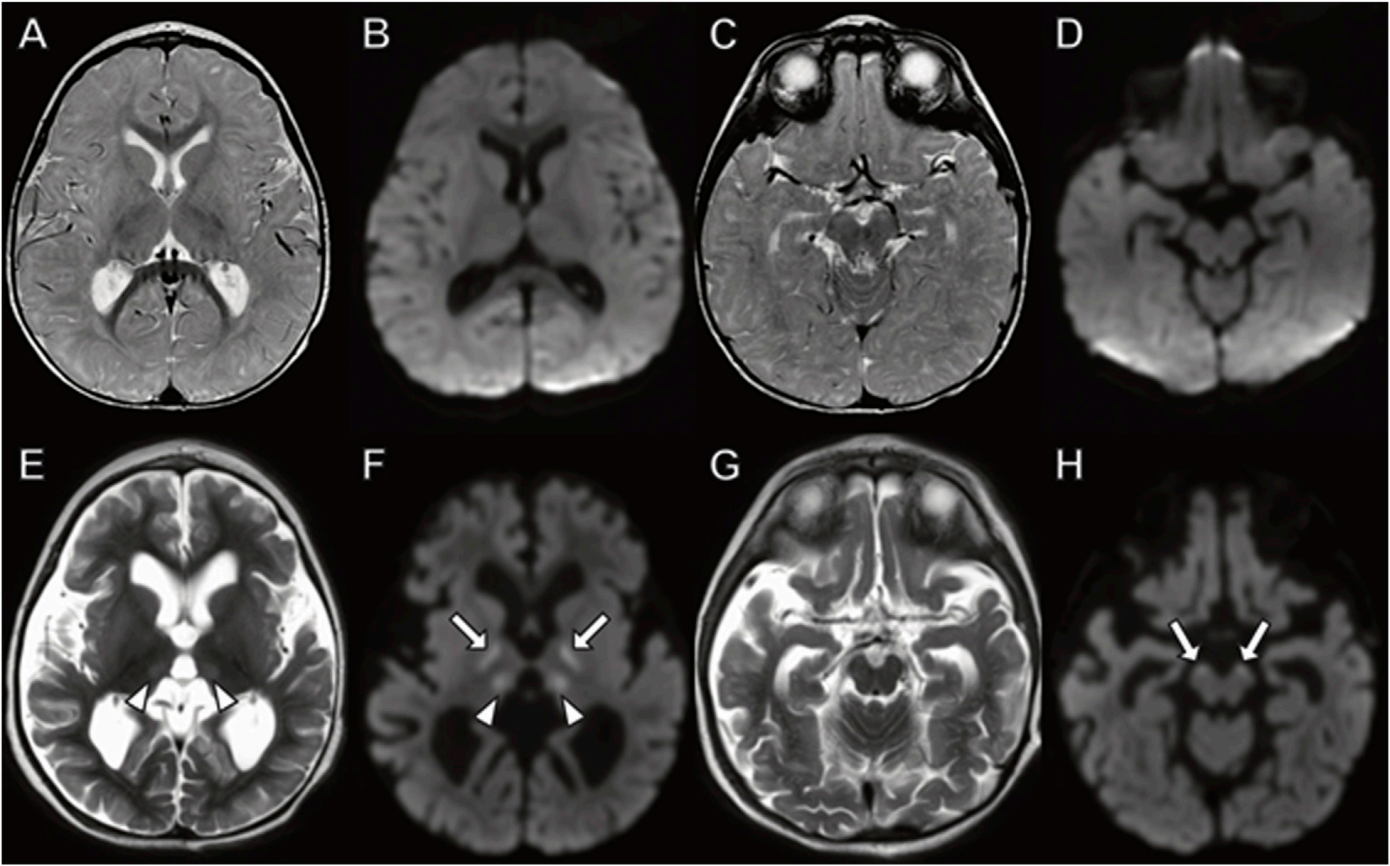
Brain MRI in boy with *TANGO2* mutation at (A–D) 8 months and (E–H) 15 months of age. The brain MRI at 8 months demonstrates no abnormal signal on (A,C) fluid-attenuated inversion recovery or (B,D) diffusion-weighted images. (E–H) At 15 months, diffuse cerebral atrophy is present with abnormal (E) T2 signal and (F) diffusion-weighted imaging hyperintensity in the thalamus (arrowheads). Additional areas of restricted diffusion involving the corticospinal tracts on diffusion weighted imaging ((F,H), arrows).
